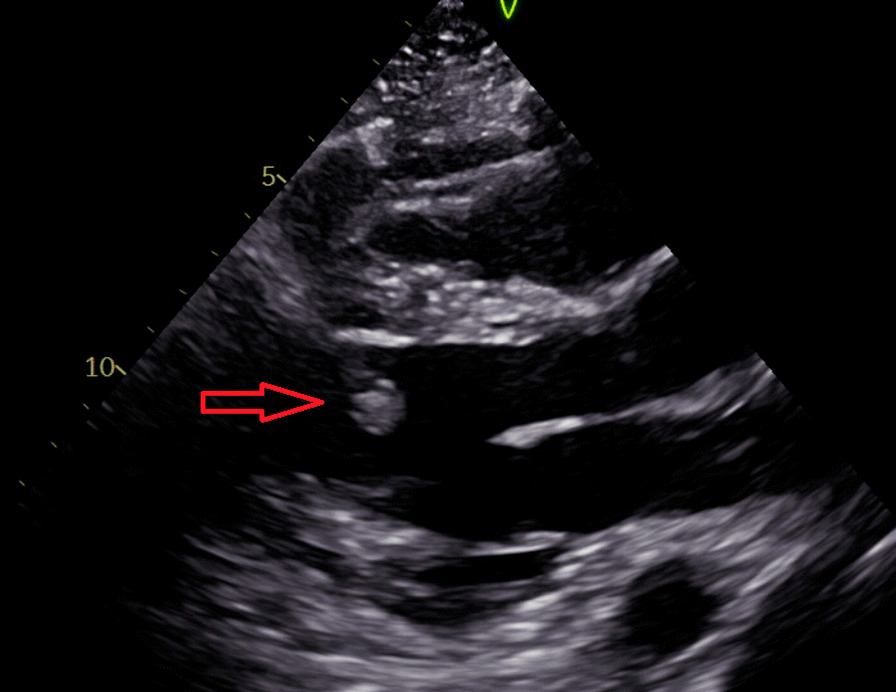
Echocardiography in parasternal long axis view performed post-operatively showing a large thrombus in the left ventricle
